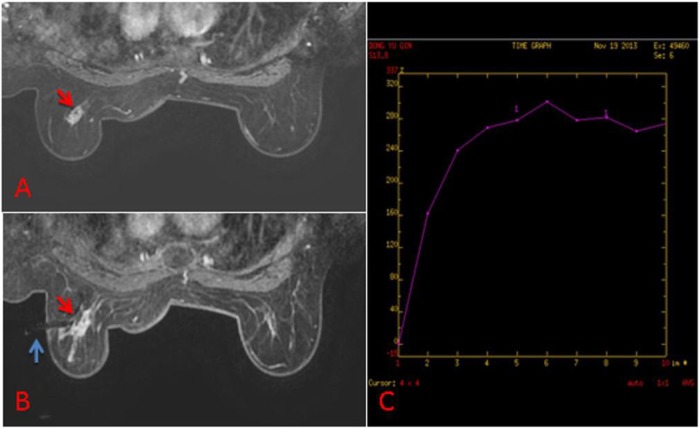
(A) 63-year old woman, T1-weighted fat suppressed contrast enhanced MRI showed a irregular mass at the left breast, which was suspicion for malignancy, and the BI-RADS category was considered as level 5. It was not found by ultrasound or mammography. (B) The lesion (red arrow) was performed by MR-guided biopsy (blue arrow), and it was confirmed to be invasive ductal carcinoma. (C) The dynamic contrast-enhanced time-signal intensity curve (TIC) was appeared as “fast-plateau type”.
